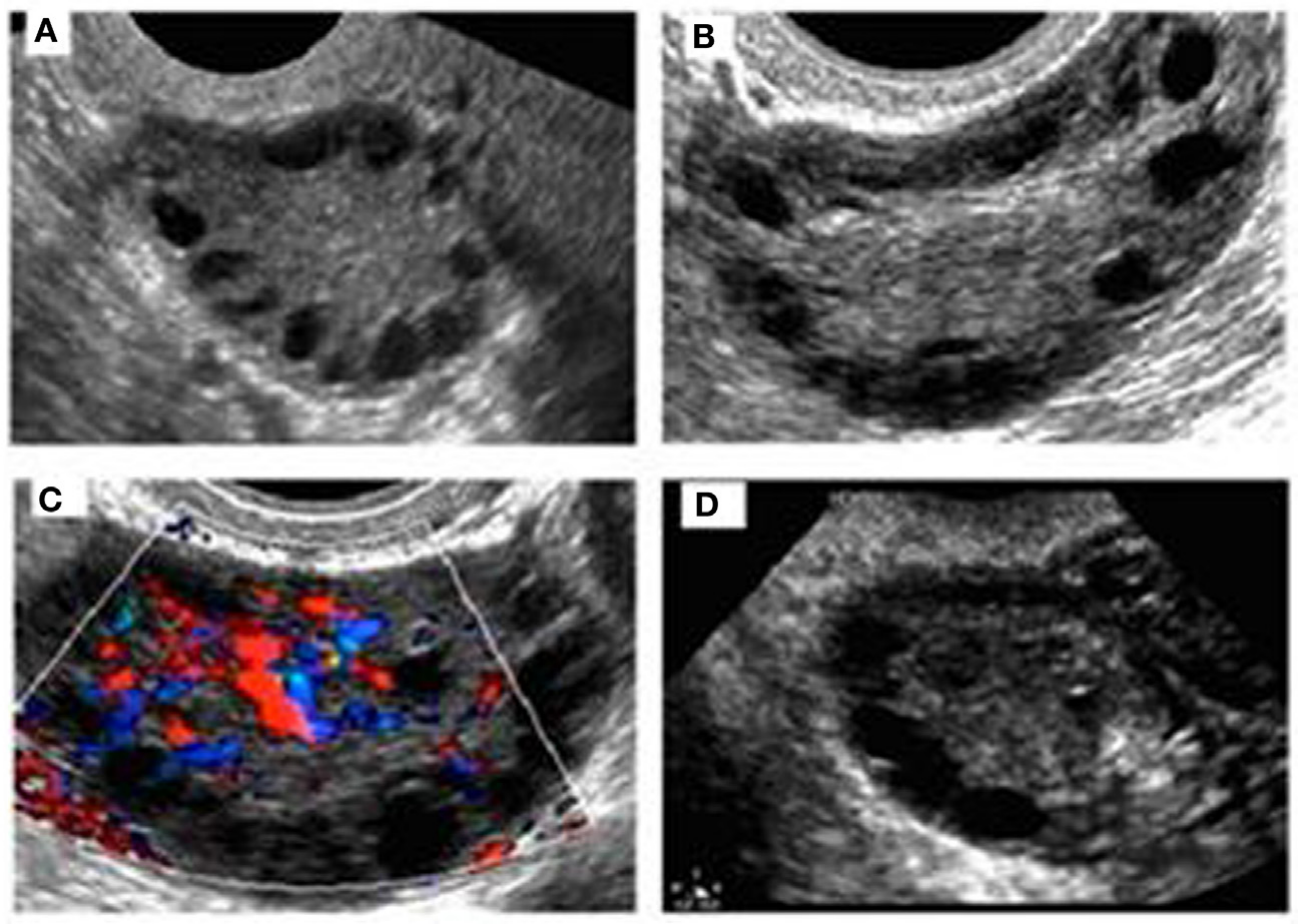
Sonographic pattern of a type A ovary (A). Note the typical rosary arrangement of follicles and the easily recognizable hyperechogenicity that results from thickening of theca (B). The stromal hypervascularity is clearly visible in (C). Secondary aspects are the dominance of follicles with a diameter of >4 mm and the predominance of longitudinal diameter (D).
